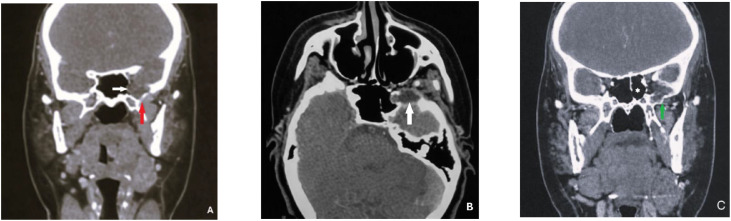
Contrast-enhanced CT of the head and neck in the parenchymal window, coronal (A) and axial (B) images, demonstrating a well-defined, smoothly marginated cystic lesion centered in the left spheno-temporo-orbital region (white arrow), extending toward the left infratemporal fossa and associated with osteolysis of the greater wing of the sphenoid (red arrow). (C) Coronal CT image in parenchymal window demonstrating well-aerated sphenoid sinuses (white star), with no communication between the mucocele and the sphenoid sinus, associated with osteolysis of the left greater wing of the sphenoid (green arrow).
